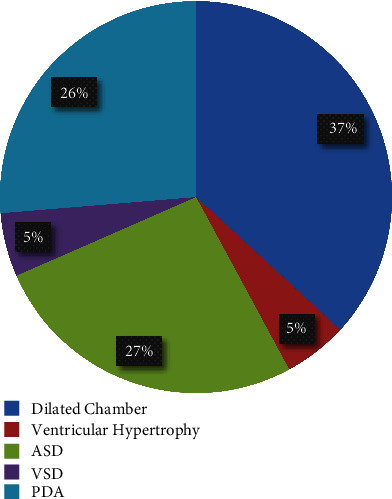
Echocardiogram abnormalities diagnosed (*n* = 20).
